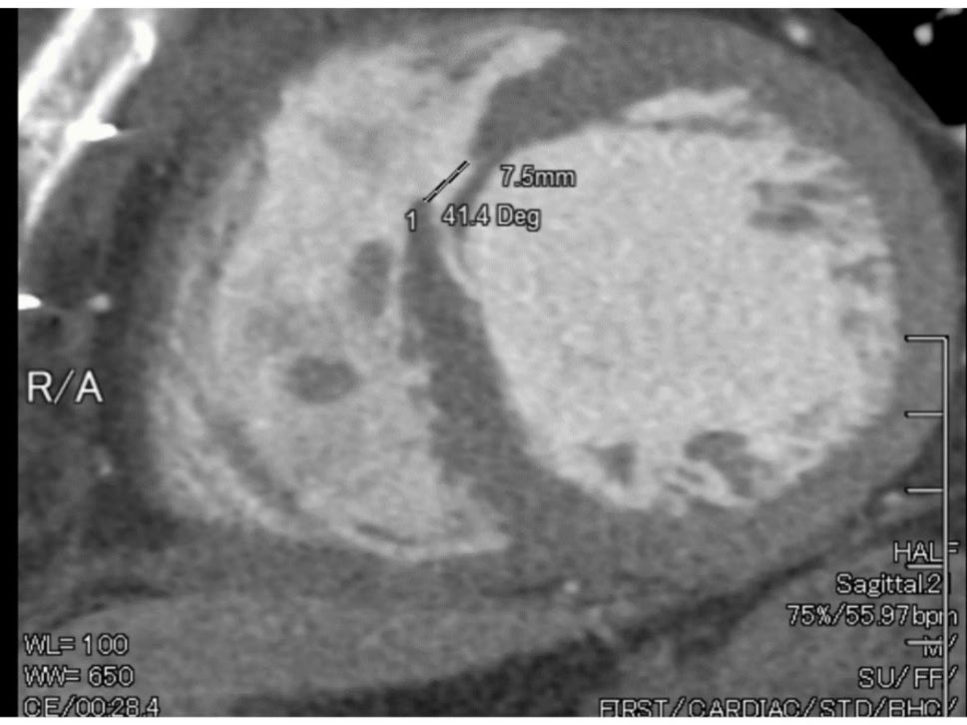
Cardiac CT Scan with contrast before the second surgery indicating VSP
